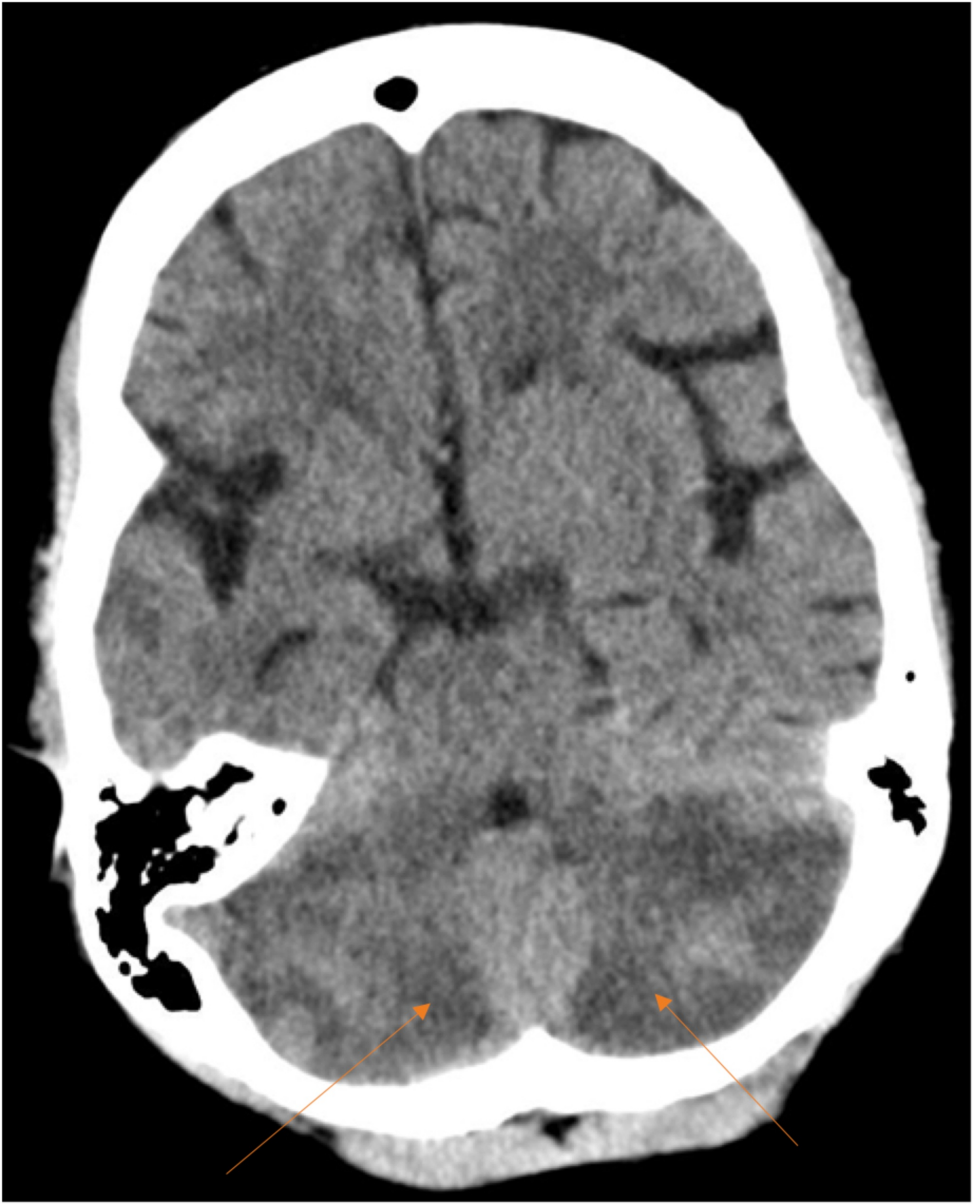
Computed tomography (CT) scan of the brain Computed tomography axial section showing bilateral posterior inferior cerebellar hemisphere hypodensity (arrows) suggestive of subacute infarction with no effacement of the fourth ventricle.
